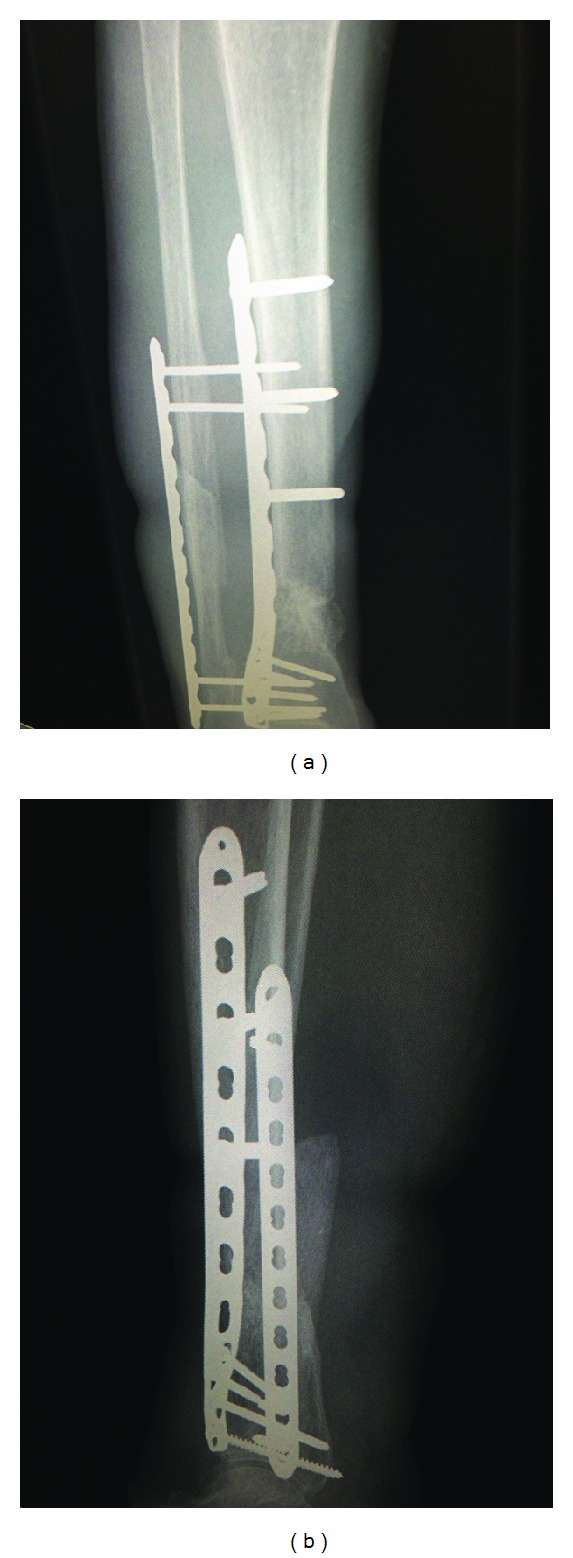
AP (a) and lateral (b) radiographs taken 6 months later showing osseous consolidation.
